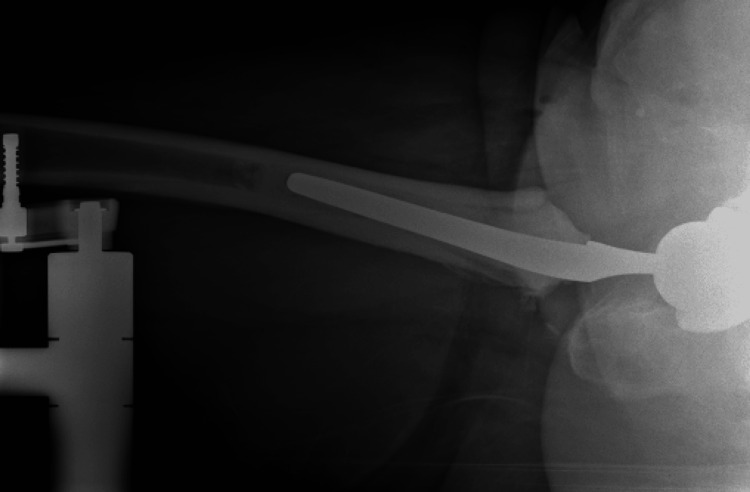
Intraoperative lateral radiograph showing the distal femoral neck cut Intraoperative lateral radiograph showing an overly distal femoral neck cut with minimal remaining neck, necessitating the use of a high-offset stem for biomechanical restoration.
